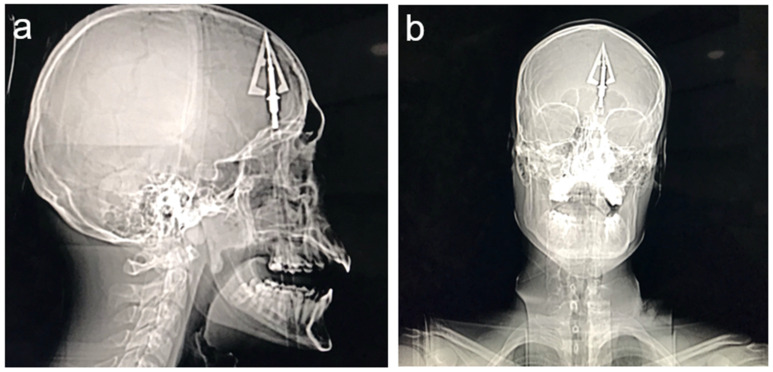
Computed tomography scan images showing the retained crossbow projectile: (a) lateral view and (b) anterior–posterior view.
